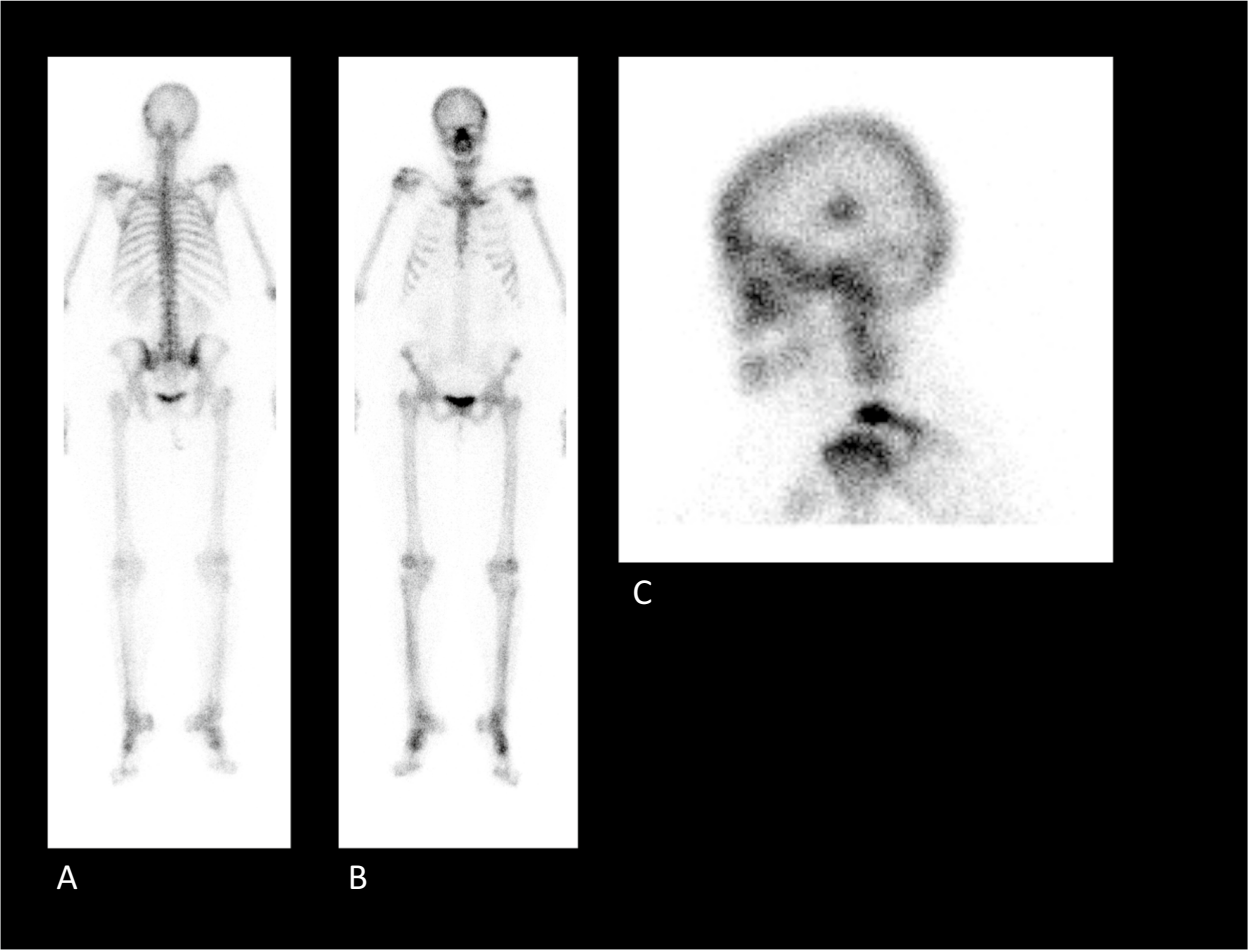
Planar skeletal scintigraphy of an FBSS patient using technetium-99m hydroxydiphosphonate (Tc-99m HDP) to investigate rheumatological disease in the tarsal and metatarsal joints. A Posterior full-body planar image; B anterior full-body planar image; C Lateral skull planar image. Although Tc-99m HDP skeletal scintigraphy was carried out to assess the tarsal and metatarsal joints, full-body planar images were also acquired. In corroboration with the findings of Huang et al. [29], planar images show no specific features of FBSS. Findings were consistent with (nonspecific) degenerative changes in the spinal column and the bilateral tarsometatarsal joints. Focal round accumulation of tracer was observed in near the left parietal bone. This incidental finding was consistent with left parietal meningioma
